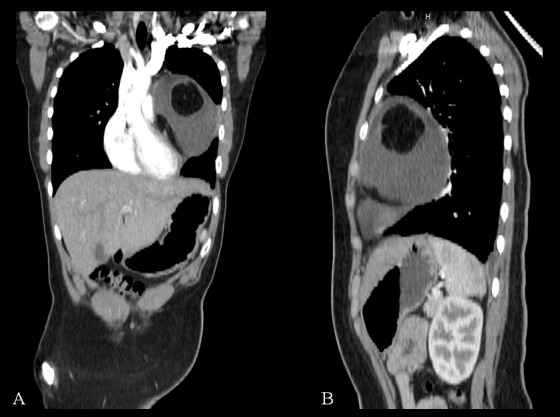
Figure 3: Coronal (A) and sagittal (B) CT reconstructions of mediastinal cystic teratoma. The mass appeared to compress the adjacent structures without invasion. A calcification is evident in coronal reconstruction.
